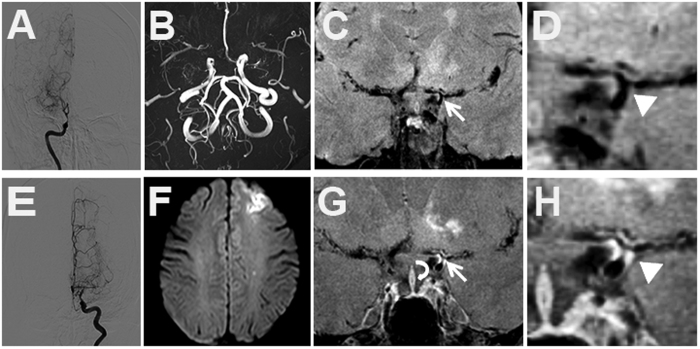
Grade 2 enhancement of MMV. (A,E) intracranial vessels on DSA. (B) Occlusion of bilateral MCAs on TOF MRA. (F) Acute ischemic infarction of left frontal lobe on DWI images. Pre- (C,D) and post-enhanced images (G,H) show stenosis in left distal ICA, proximal MCA and ACA (white arrow and arrow head). The enhancement in the bifurcation of left distal ICA is greater than that of pituitary infundibulum (curved arrow).
